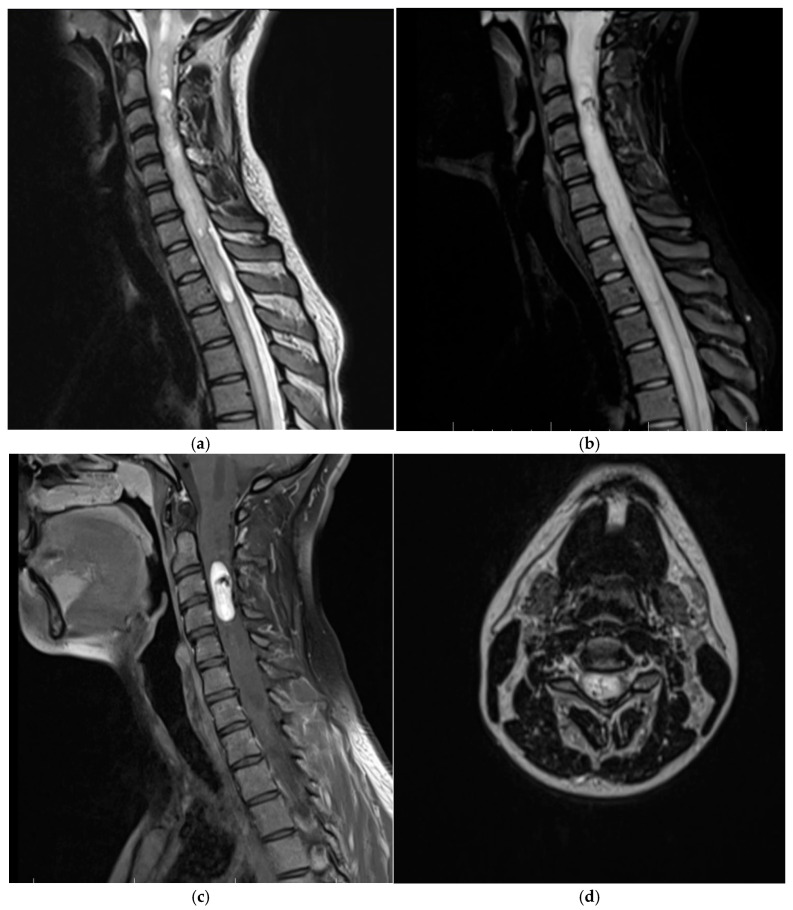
MRI preoperative exam of patient two revealing a cervical medullary hemangioblastoma. (a) Sagittal section, T2 sequence, which reveals a heterogeneous tumor process located intramedullary cervical, with isointense and hyperintense areas, having non-red flow void signals, due to dilated vessels, medullary edema and syringomyelia. (b) Sagittal section, T2 TIRM sequence, revealing marked perilesional marrow edema. (c) Sagittal section, postcontrast T1 sequence, which highlights an intramedullary tumor formation, located posterior to the C2 and C3 vertebral bodies, with intense and heterogeneous contrast uptake. (d) Axial section, postcontrast T1 sequence highlights the intermedullary location of the tumor.
